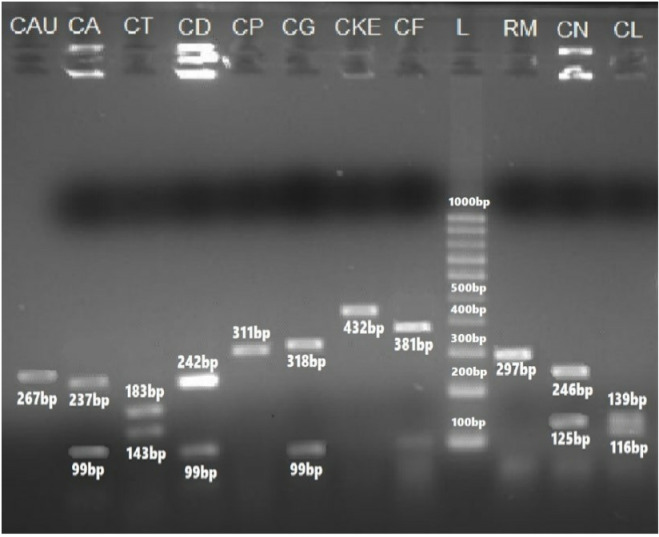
Gel image of PCR-RFLP assay using *Msp*1 as a restriction enzyme for the digestion of PCR products ITS3&4 of CAU= *Candida auris* (267 bp), CA= *C*. *albicans* (237 bp, 99 bp), and CT=*C*. *tropicalis* (183 bp, 143 bp), CD= *C*. *dubliniensis* (244 bp, 99 bp), CP= *C*. *parapsilosis* (350 bp, 200 bp), CG= *C*. *glabrata* (318 bp, 99 bp), CKe= *C*. *kefyr* (432 bp) CF= *C*. *famata* (381 bp), CN= *Cryptococcus neoformans* (246 bp, 125 bp) CL= *C*. *lusitaniae* (139 bp, 116 bp) as compared to 100 bp DNAladder (L).
